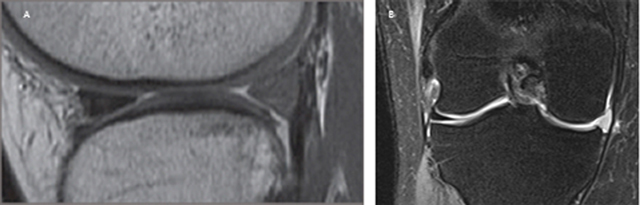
(A) Sagittal T1-weighted fast spin echo MR-image demonstrating the slightly hyperintense polyurethane scaffold at the posterior horn of the lateral meniscus. (B) Coronal fat saturation T2-weighted fast spin echo MR-image demonstrating a hyperintense polyurethane scaffold in the medial compartment. The native meniscal rim can be seen as a black rim encapsulating the scaffold. The scaffold demonstrates moderate radial displacement.
